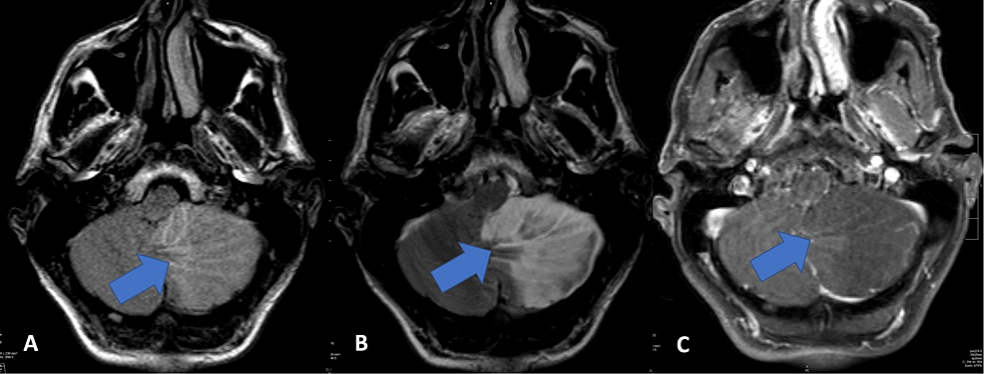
Axial fluid-attenuated inversion recovery (FLAIR) (A), T2 (B), and axial T1 (C) post contrast series reveal widened cerebellar folia with a striated/laminated appearance in the left cerebellum without contrast enhancement.
